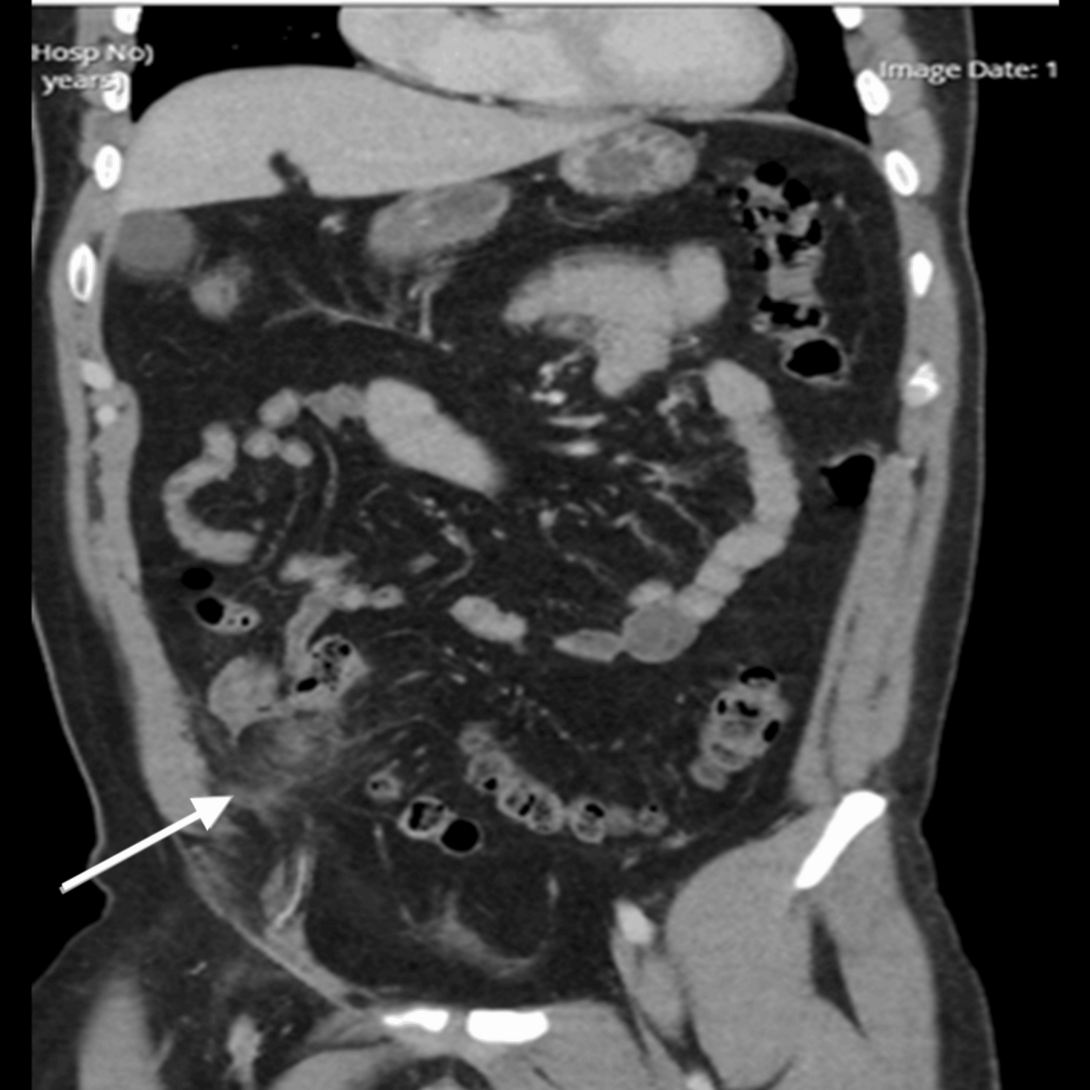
Coronal CT image demonstrating the same lesion in the right iliac fossa, with preserved appendix.
